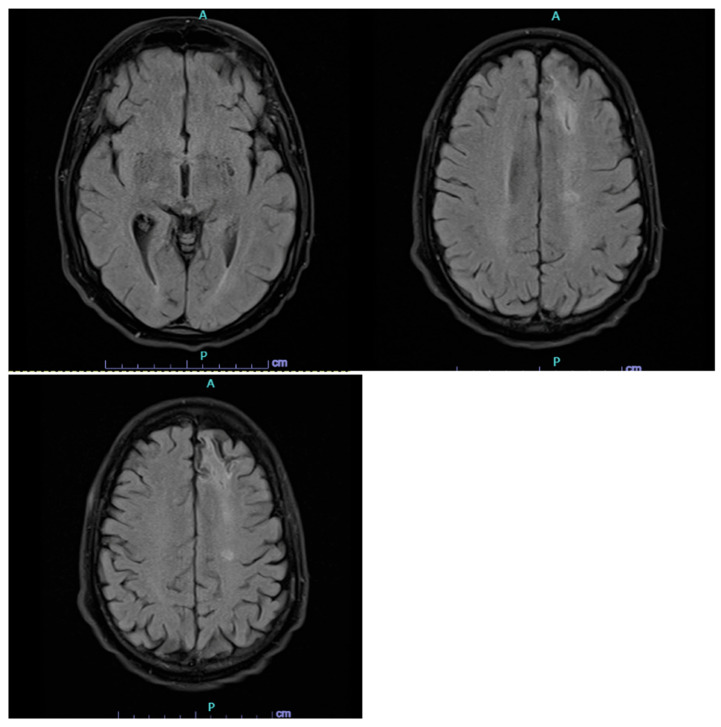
T2 fluid attenuated inversion recovery magnetic resonance images of the brain from most inferior to most superior with evidence of the new left-sided acute infarct and left-sided frontal lobe encephalomalacia. There is no evidence of encephalitis or inflammation on this image set.
